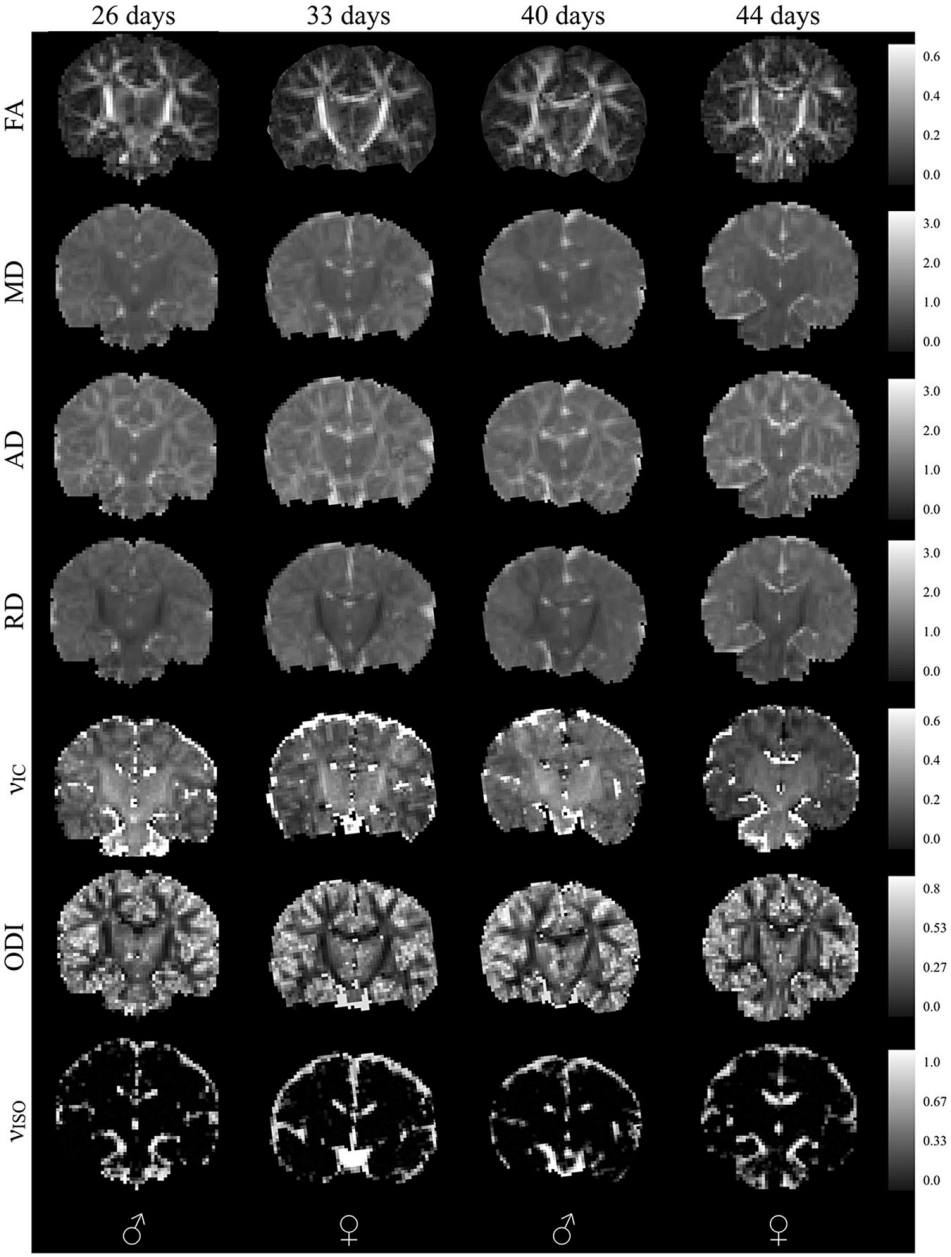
Coronal raw DTI (FA, MD, AD, and RD) and NODDI (ν_IC_, ODI, and ν_ISO_) estimates from four representative infants. The sex of the infant is denoted in the bottom panel. The optimized diffusion protocol resulted in high quality DWI data and parameter maps.
